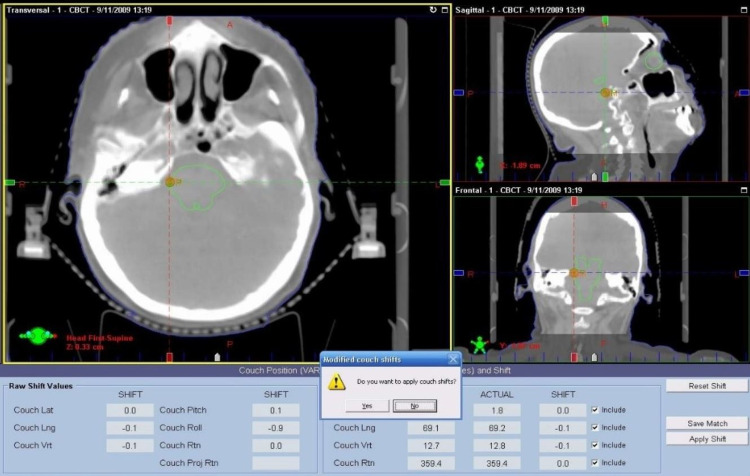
Following initial ExacTrac positioning, CBCT is obtained for independent confirmation of patient position. The patient is then stereoscopically imaged prior to the delivery of each arc and repositioned when 0.5-mm translational tolerance in any direction is exceeded. CBCT, cone beam computed tomography
